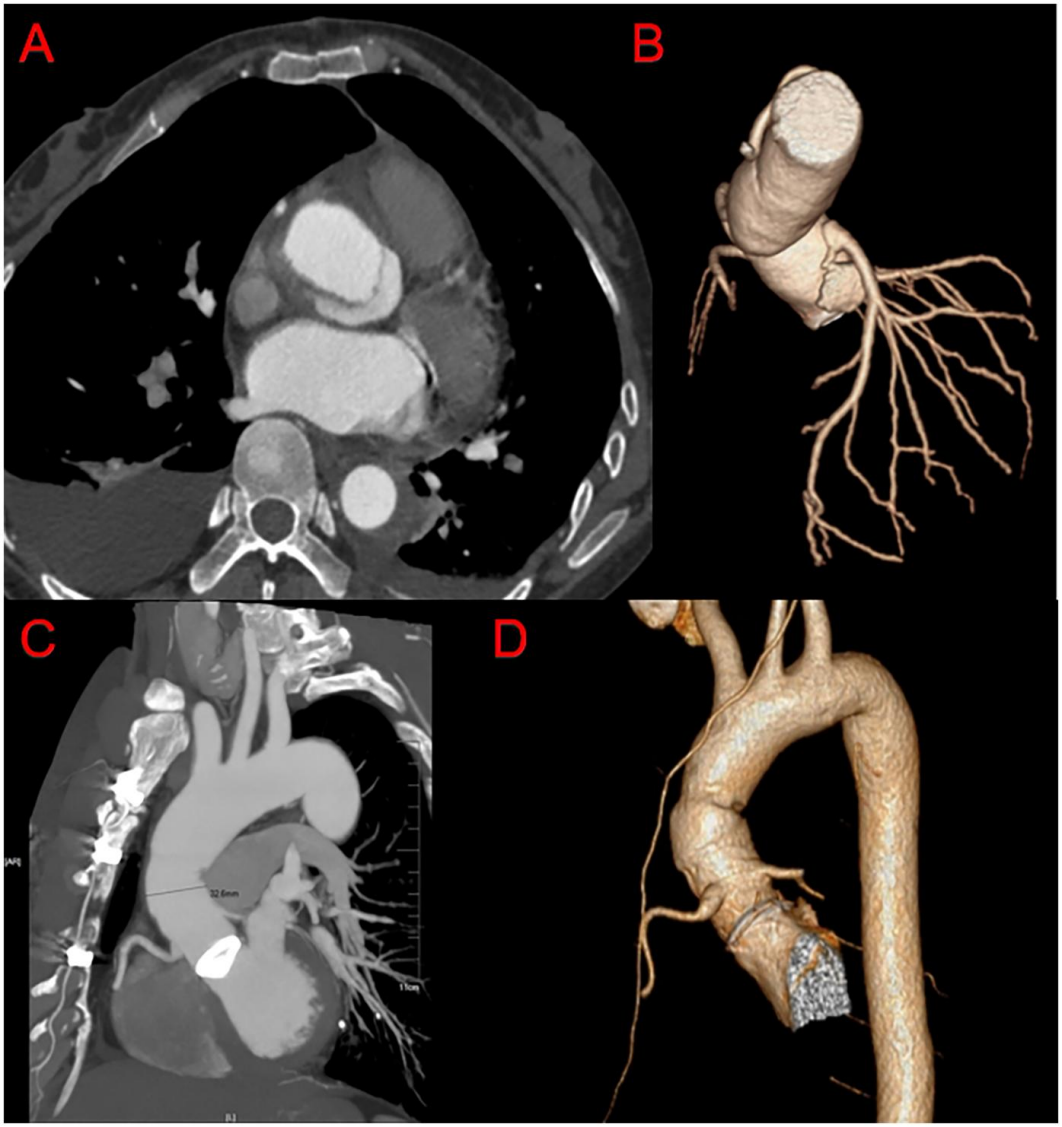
Post-operation CTA imaging of our modified bentall procedure. (A,B) Follow up CTA of the patients with the diameter of aortic root enlargement. (C,D) Follow up CTA.
